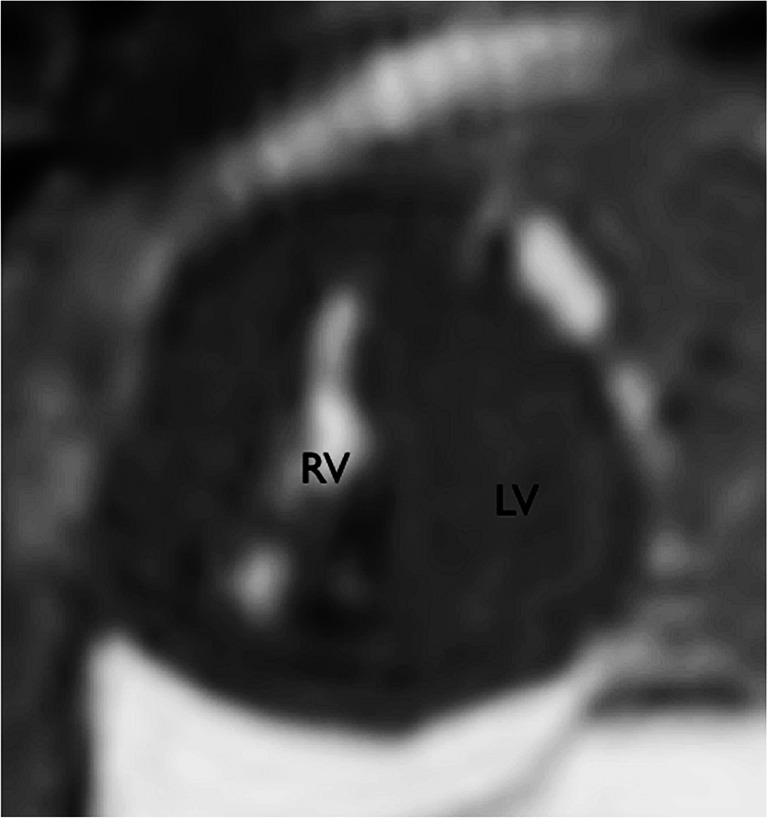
Fetal cardiac postmortem MRI shows hypoplastic left heart syndrome on sagittal T2-weighted image in a 22-week gestation fetus. *LV* left ventricle, *RV* right ventricle. Reproduced with permission [14]
